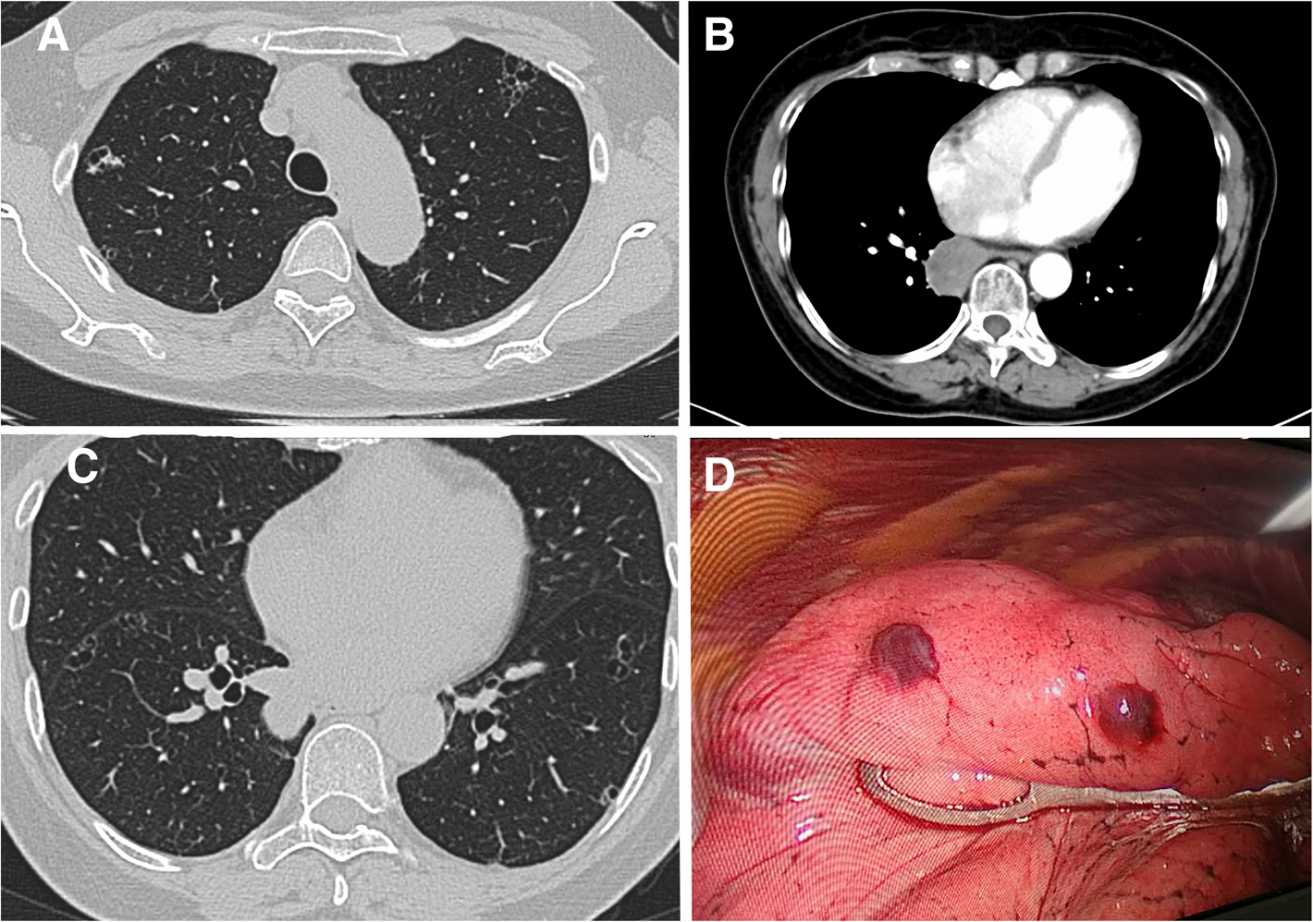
a, c: CT revealed multiple cycstic lesions involving both lungs. b: Major mass located in the right posterior mediastinum. d: Lung biopsy was taken for pathological examination
